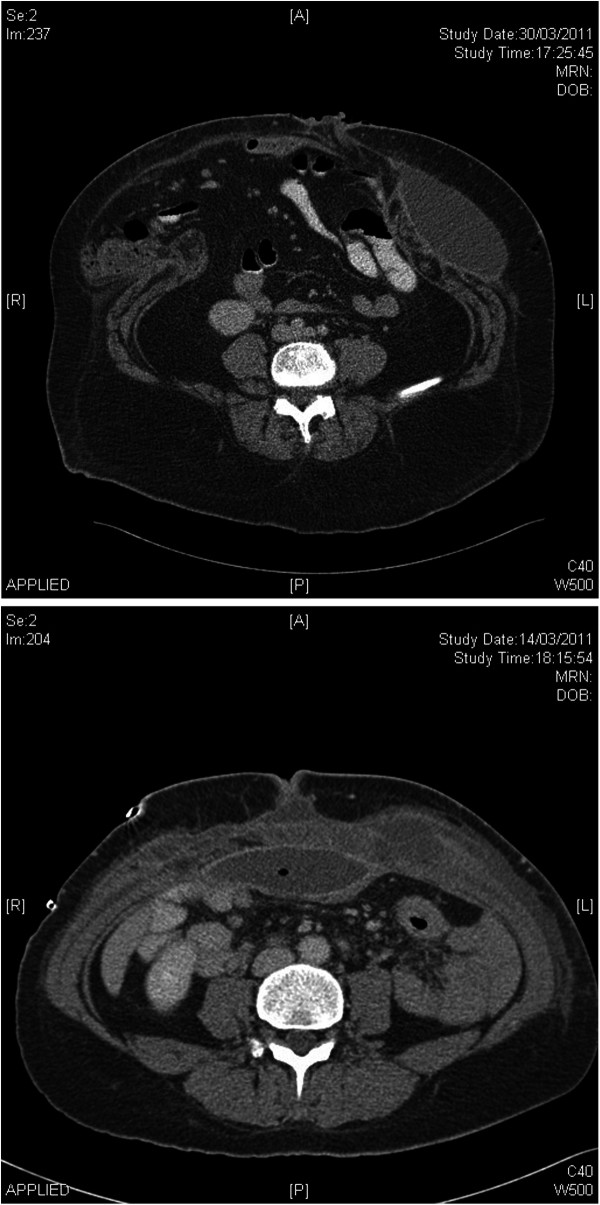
CT scan images of (i) Top image; complete rupture of an incisional hernia repair; (ii) Bottom image; Seroma formation above and below a sublay mesh repair.
